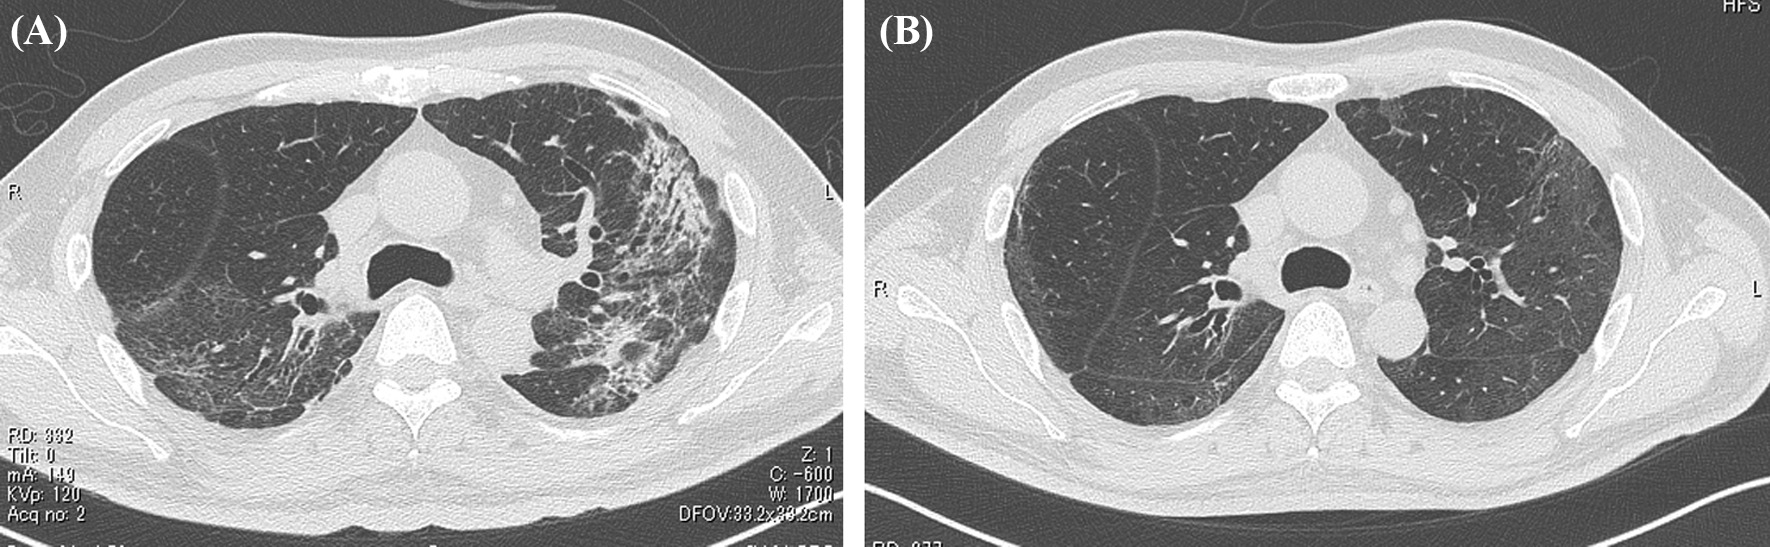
Computed tomography image of the chest. A Day T + 30. Note the resolution of the shadows in the bilateral lower lobes, with a band of infiltrating shadows remaining at the periphery of the bilateral upper lobes. B Day T + 78. Note the resolution of the band of infiltrating shadows. Figure is taken from Kashiwabara* et al.* [5], with permission
